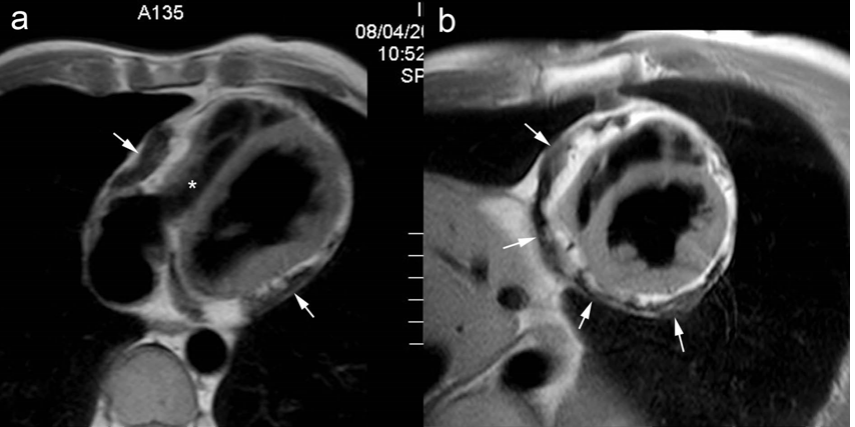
Classic presentation of constrictive pericarditis on T1-weighted fast spin-echo CMR, axial view (a), and short-axis view (b). Extensive pericardial thickening is found on the laterobasal and inferior part of both right and left ventricle and atrioventricular grooves (arrows). The thickened areas are irregularly defined, and are compressing the adjacent cardiac cavities, mainly on the right (*).
